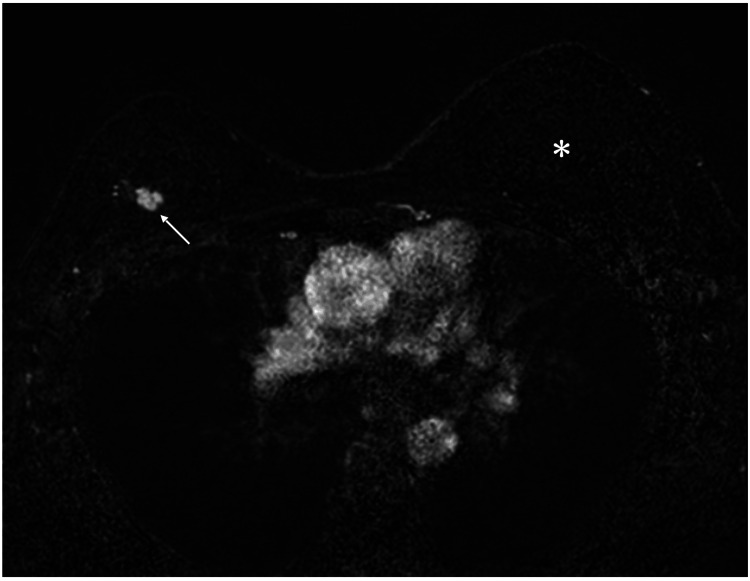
MRI findings MRI showed that the right breast was smaller than the reconstructed left breast (asterisk) after radiofrequency ablation and radiotherapy, in addition to the fast enhancement (arrow) of the right breast cancer. MRI: magnetic resonance imaging
